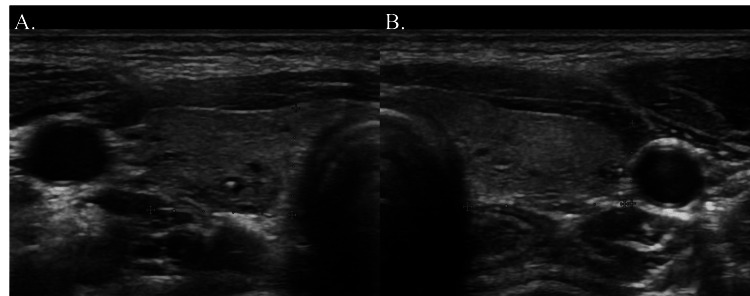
Ultrasound image of the thyroid of the patient (A) Right lobe of the thyroid. (B) Left lobe of the thyroid. The parathyroid glands were not observed.
